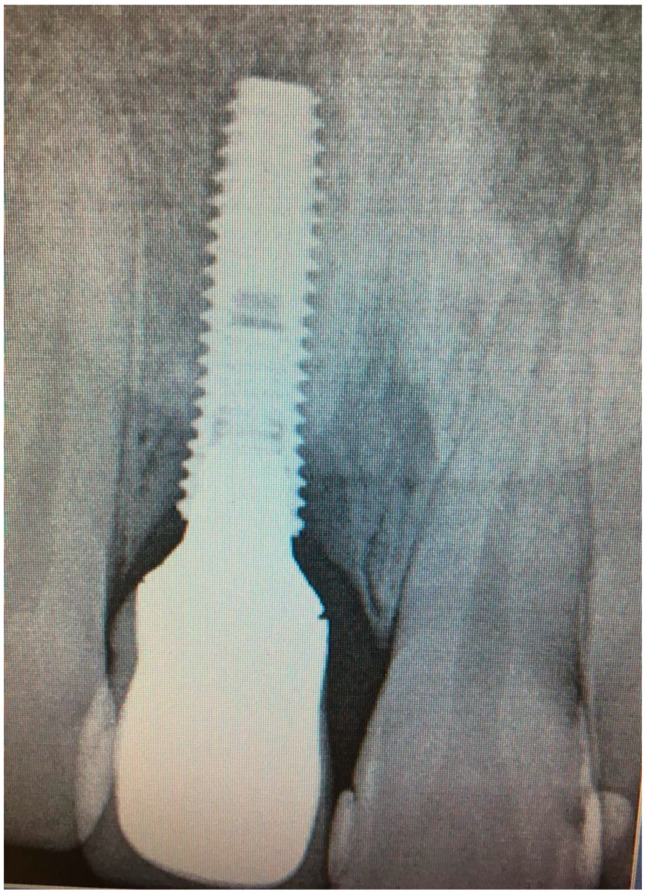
X-ray of patient in 6 months after treatment.
